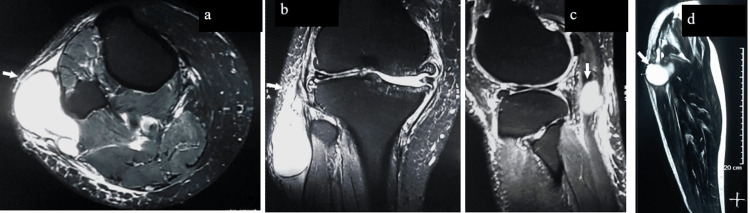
The MRI of the right knee shows an axial T1-weighted image (a), which demonstrates a uniformly hypointense mass (arrow), a hypointense lesion (b) on the coronal MR image, and a sagittal T2-weighted image (c) demonstrating a tubular bright cystic mass (arrow) just posterior to the tendon, as well as a perineural extra-articular cyst on the posterolateral aspect of the knee (d)
